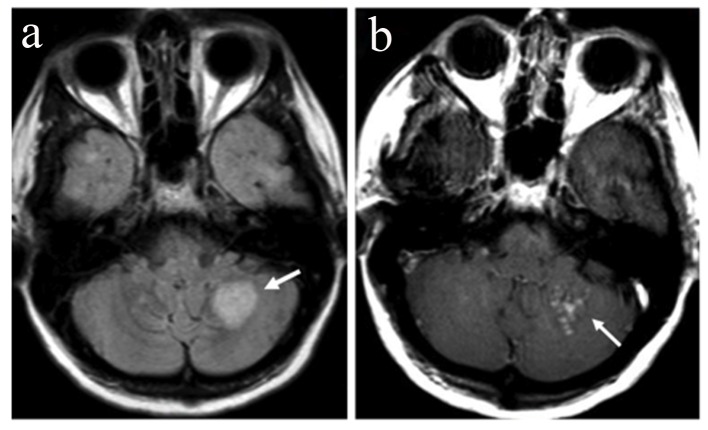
On month 4, MRI demonstrates a new 2-cm lesion in the left cerebellar hemisphere with high signal on FLAIR (arrow, a) and a speckled pattern of contrast enhancement on the post-gadolinium image (arrow, b).
